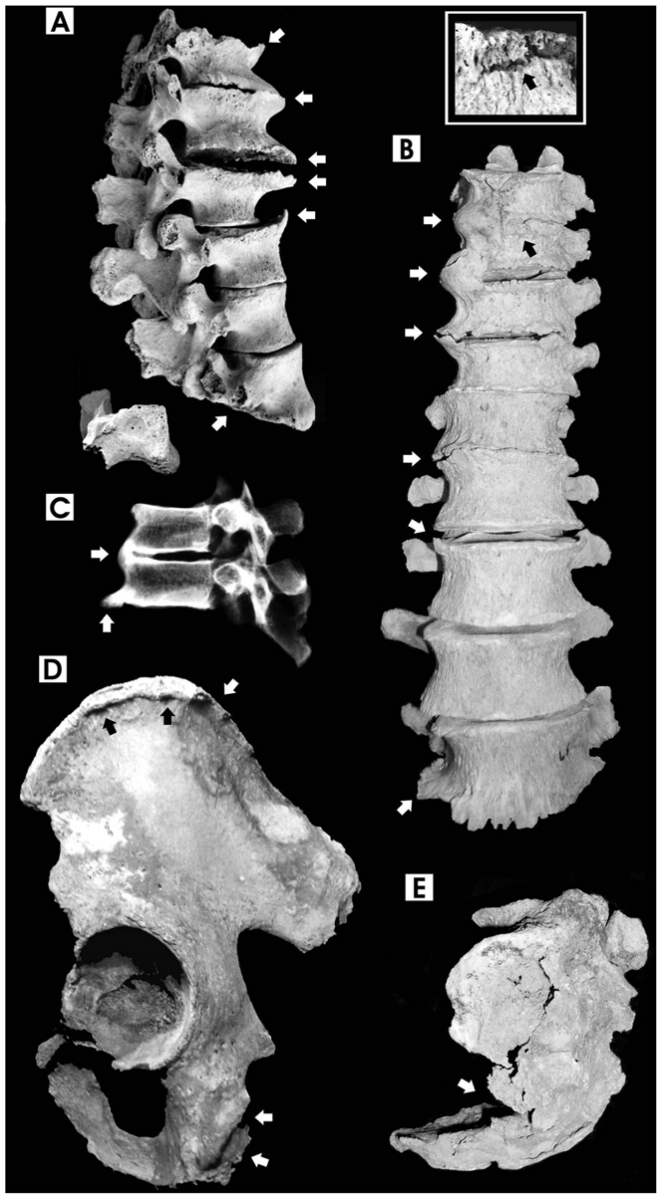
Pathological features of spine and pelvis. A. Widespread hypertrophic osteosclerosis, calcification of anterior ligaments, spondyloarthrosis and osteoporosis (arrows) of thoraco-lumbar vertebrae (T12-L5, lateral view), 44-year-old male. Notice severe flattening (osteoporosis) of the L5 vertebral body (arrow) and lumbar spondylolysis (inferior articular part split separately from the spinous process); B. Healed fracture of T10 (see enlargement in the small box), severe calcification of thoraco-lumbar anterior ligaments (T9-L5, anterior view) and ankylosis of T9-T11 vertebrae (arrows), 52-year-old male. Spondylolysis affects the L5 vertebra too; C. Digital X-ray image of T8-T9 fused vertebrae, showing diffuse osteosclerosis (lateral view), 38-year-old male; D. Ligamentous calcification and osteophytic bony spurs at the iliac crest and ischial tuberosity (sacrotuberous ligament) (arrows), 52-year-old male; E. Healed fracture of the 3^rd^ vertebra (arrow) and kyphosis of the sacrum bone (lateral view), 52-year-old male (bone images are in 1∶2 size).
